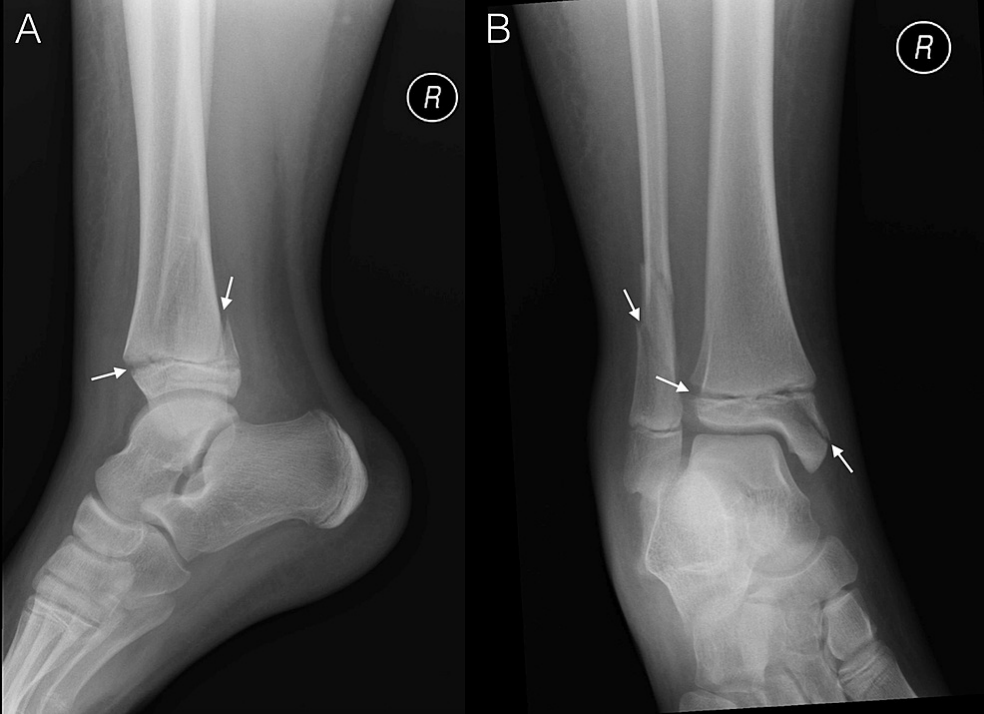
Anteroposterior and lateral x-rays of the right ankle. The radiographs demonstrate a spiral fracture of the distal fibula, mildly displaced fractures of the posterior and medial malleoli, and a degree of physeal widening anterolaterally.
